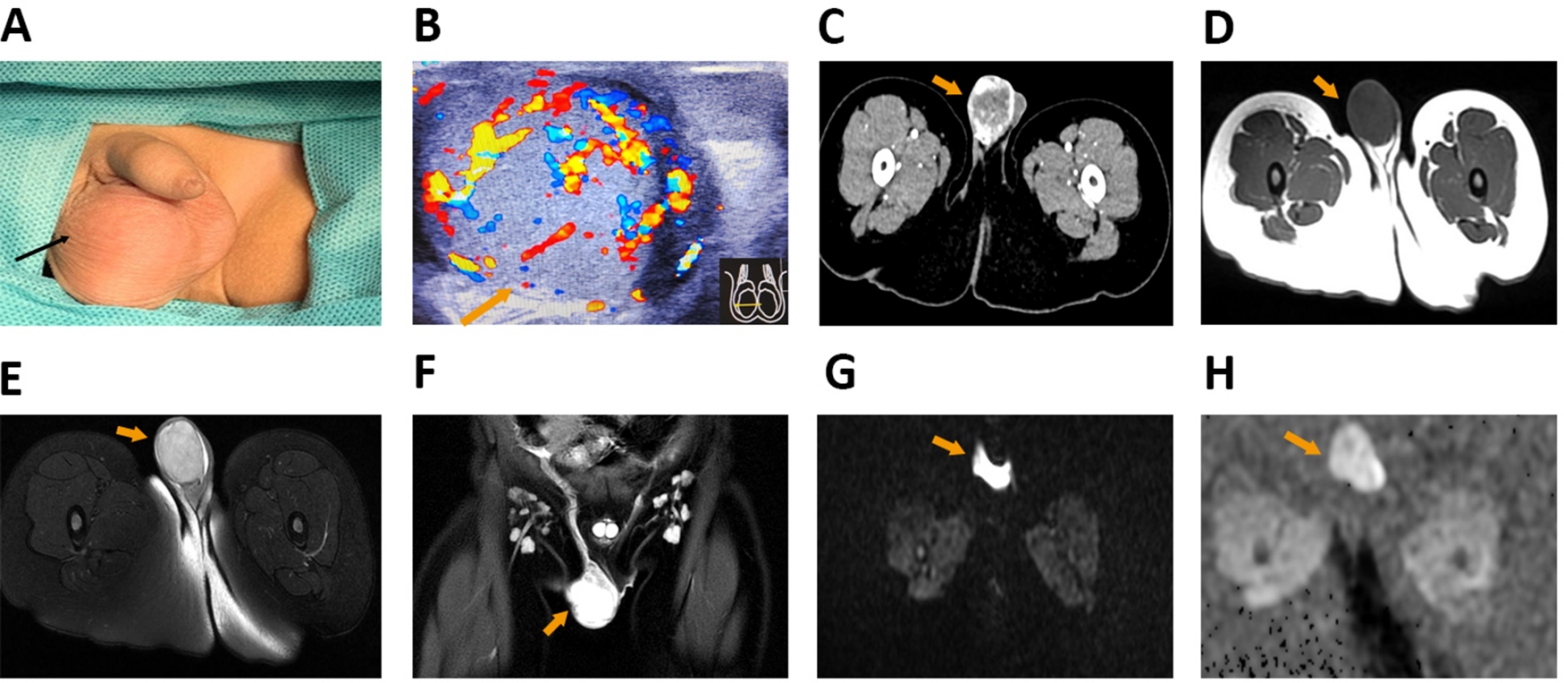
The results of preoperative examination. (A) The physical examination showed right scrotal painless and rubbery swelling. (B) The results of perineal color Doppler ultrasonography showed that the size of right testis was 29 mm × 22 mm × 20 mm, the PSV was 15.4 cm/s, and RI was 0.65. (C) CT showed the right testis with space-occupying lesions. (D–H) MRI showed slightly equal SI on T1WI (D), high SI (E) and homogenized enhancement of enlarged multiple lymph nodes in bilateral inguinal region (F) on T2WI, insignificant diffusion restriction on DWI (G), and high SI on ADC (H) in the lesion of right testis. PSV, peak systolic velocity; RI, resistive index; SI, signal intensity; T1WI, T1-weighted imaging; T2WI, T2-weighted imaging; DWI, diffusion-weighted imaging; ADC, apparent diffusion coefficient.
